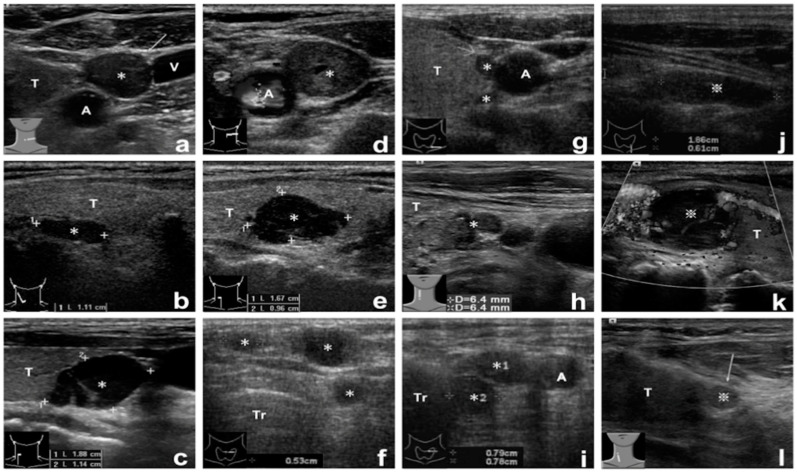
Images of positive and suspicious parathyroid sonography lesions. (a–c) Case 1, 2, 6, with positive sonographic lesions. (d) Case 5, with suspicious left upper neck lesion. (e) Case 20, with suspicious intrathyroid lesion. (f) Case 24, with suspicious multiple subcutaneous lesions. (g) Case 25, with suspicious dumbbell shape lesion. (h) Case 8, with suspicious infrathyroid lobulated lesion. (i) Case 26-1, with suspicious multiple paratracheal lesions. (j) Case 14, with suspicious intrathymus lesion (false assay-negative). (k) Case 31, with suspicious intrathyroid lesion. (l) Case 26-2, with suspicious infrathyroid small lesion. ✻ Positive tissue aspirate PTH assay lesions. ※ Negative tissue aspirate PTH assay lesions. Demographic data of enrolled patients are listed in Table S1. Abbreviations: A, common carotid artery; V, internal jugular vein; T, thyroid; Tr, trachea.
